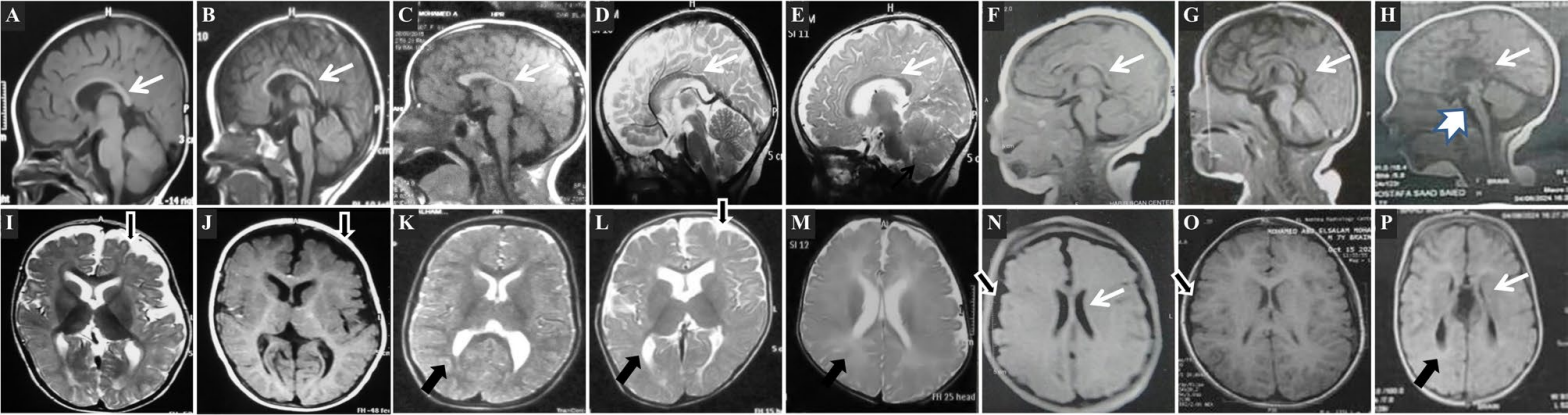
Patients’ MRI: Sagittal view cuts (A-H) showing corpus callosum abnormalities *(thin white arrow)*: thin corpus callosum (A, B, D, E), dysgenesis of corpus callosum (C, F, G), absent corpus callosum (H) and mild hypoplastic vermis and brainstem (H) *(thick white arrow*,* P24*). Axial view cuts (I-P) showing mild cortical atrophic changes (I, J, L, N, O) *(black arrow with a white edge)*, deep white matter changes (K, L, M, P) (*black arrow*), colpocephaly and agenesis of corpus callosum (P) *(white arrow)*, mild dilated lateral ventricles (I, K, L, M).
